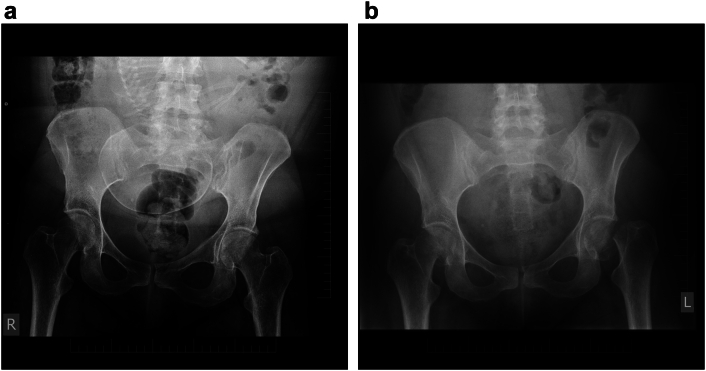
Pelvic radiograph of a pregnant patient with transient osteoporosis of the hip and fractures as a complication of treatment. 1a, - Radiograph demonstrating no pathological findings on initial imaging at admission. 1b, - Radiograph demonstrating bilateral subcapital femoral neck fractures identified postpartum.
